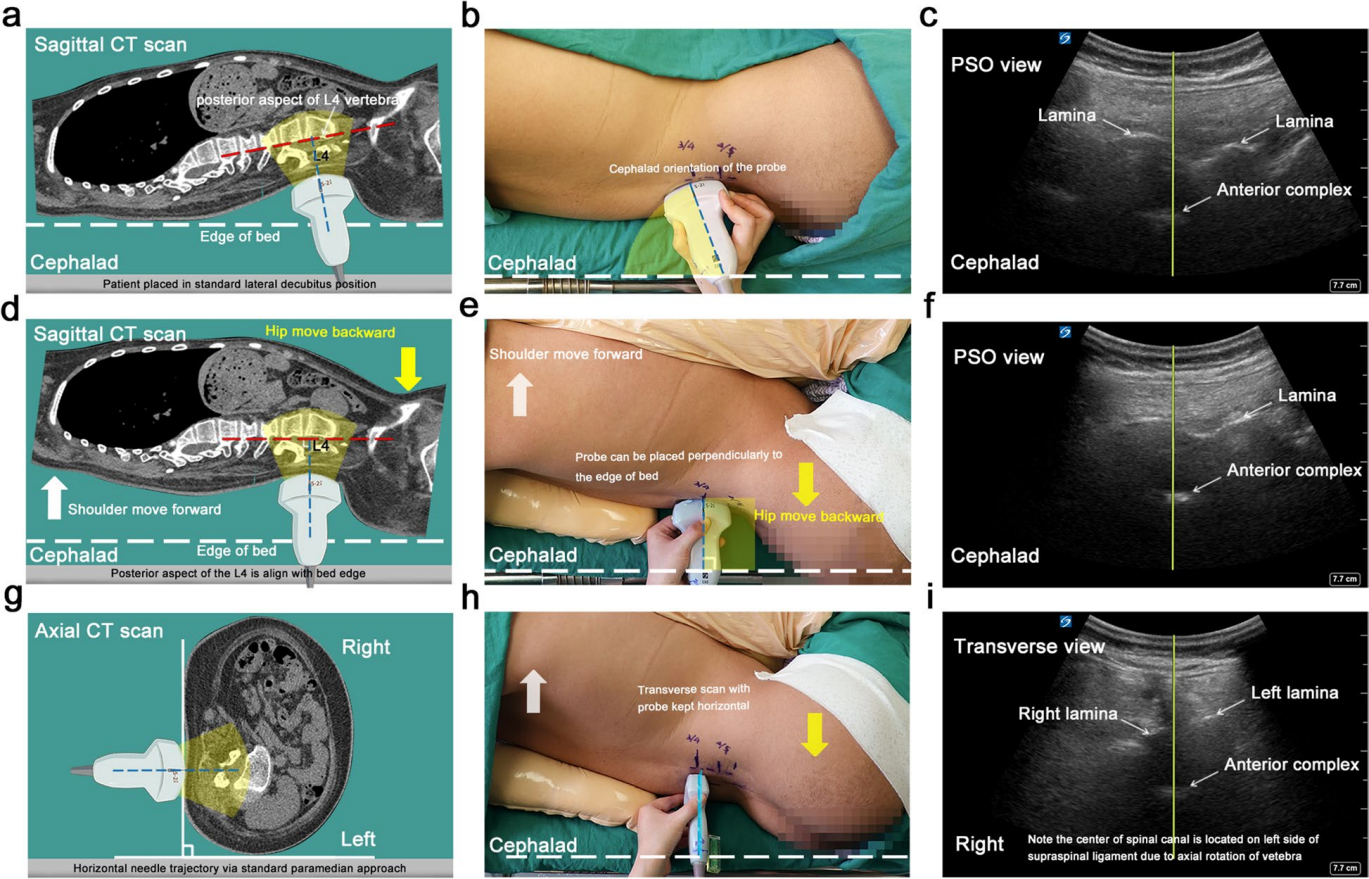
Posture correction guided by the orientation of the ultrasound probe. a Sagittal CT view demonstrating the posterior aspect of the L4 vertebra is oblique under standard lateral decubitus position, with the shoulder and the hip being placed at the edge of the bed. b-c Note the orientation of the probe relative to the edge of the bed (cephalad direction) to obtain the clear anterior complex image in the paramedian sagittal oblique view. d Sagittal CT image demonstrating the posterior aspect of the L4 vertebra being adjusted to align with the edge of the bed following posture correction. e–f Modified position with the shoulder moved forward to eliminate the cranial angulation of the probe is required for the clear anterior complex image, ensuring that the needle enters the spinal canal perpendicularly to the edge of the bed. g Axial CT view demonstrating the potential horizontal needle trajectory via the standard paramedian approach. h-i Note the probe being placed perpendicularly to the edge of the bed and horizontally, with the spinal canal centered. The blue dashed lines indicate the needle trajectory. The white dashed lines indicate the edge of the bed. The white arrow indicates moving the shoulders forward, away from the edge of the bed and the yellow arrow indicates movement of the hips backward, towards the edge of the bed
